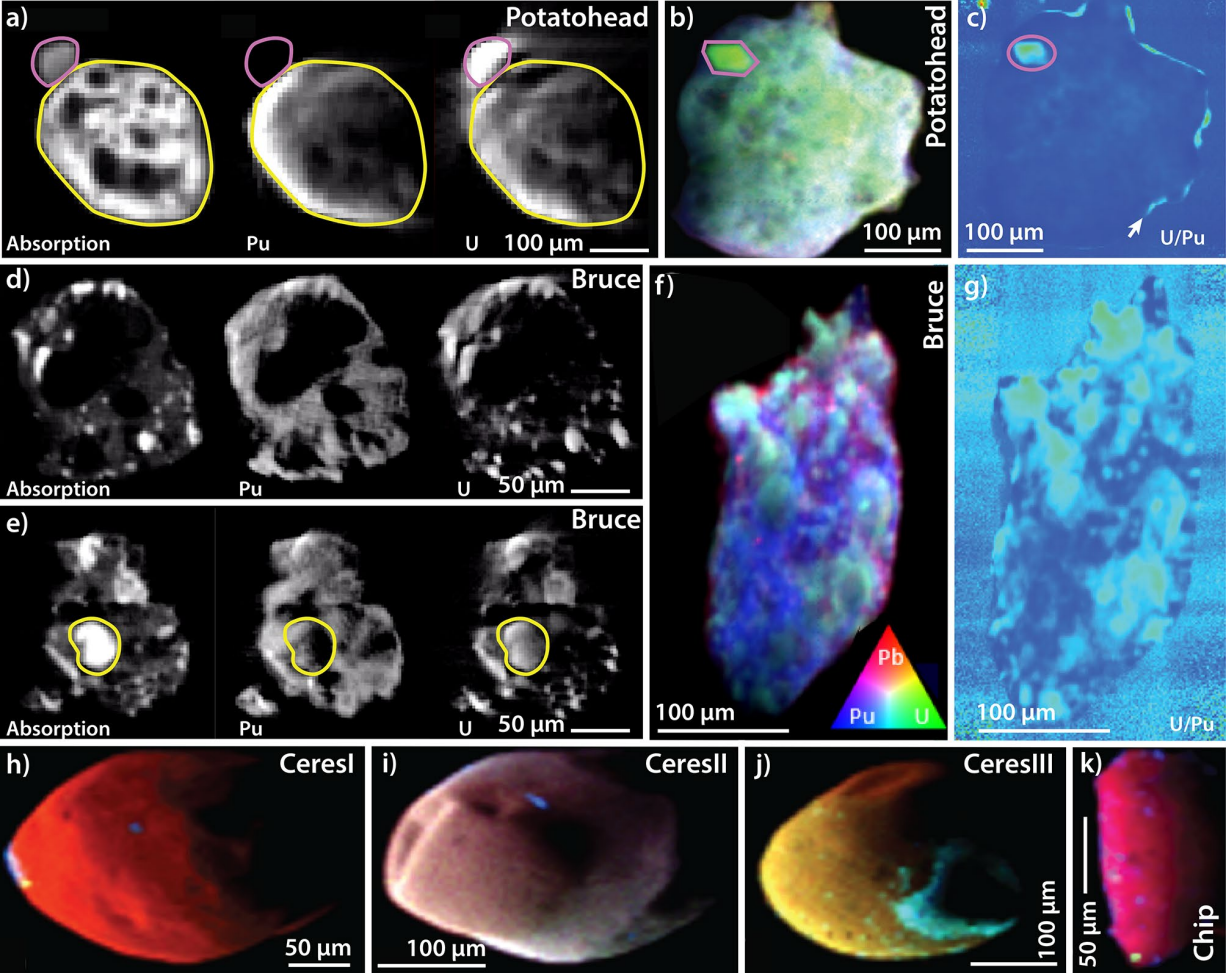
X-ray µ-tomography (a,d,e) and µSXRF imaging (b–c, f–i) of hot particles from Maralinga. In (a) (*Potatohead*) the low-X-ray cross-section areas within the particles (black) correspond to pores, whereas in (d) and (e) (*Bruce*) they correspond to Al-oxide rich domains. *Potatohead* displays a U-rich grain (purple circle in a,b,c) attached on the outside of the particle; in (e), a U-rich, Pu-poor inclusion is highlighted in yellow.
